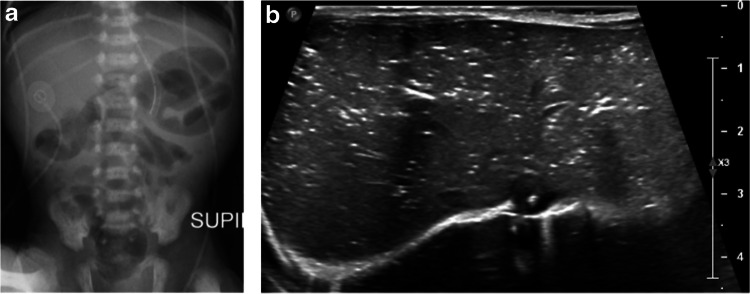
A 5-day-old male born at 32 0/7 weeks (case 2 in Table 4). Ultrasound imaging upgraded modified Bell’s stage from none to stage 2B. a An AP supine (SUPI) abdominal radiograph suggests mild separation of bowel loops but no distension, obstruction, or definitive pneumatosis. b Transverse ultrasound image of the right upper quadrant performed 31 min later reveals extensive portal venous gas throughout the liver
